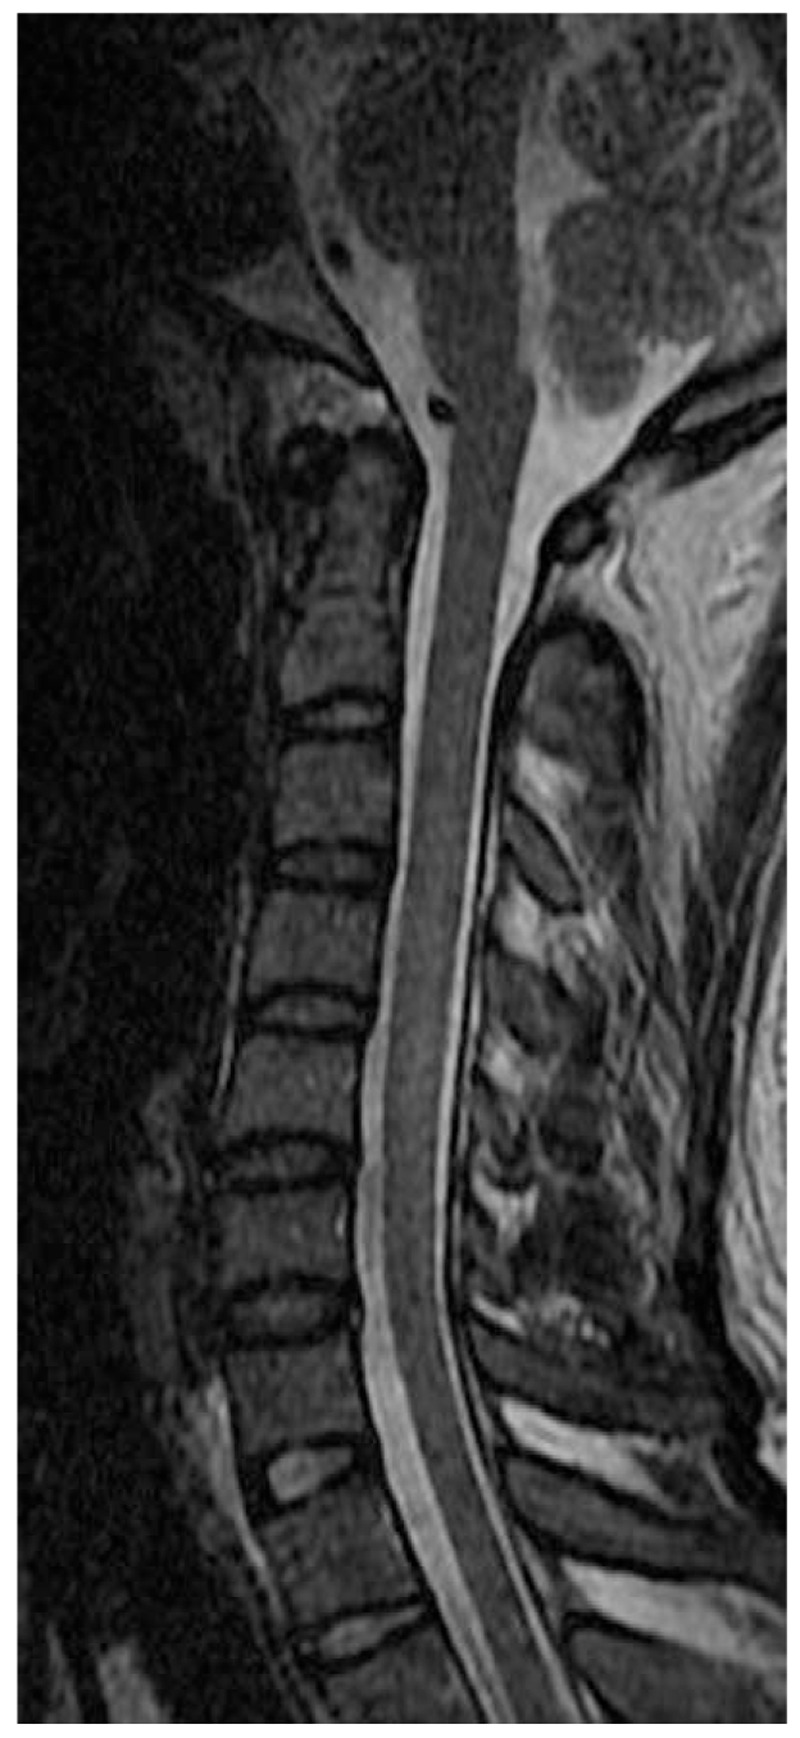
Sagittal magnetic resonance imaging demonstrates increased T2 signal in the posterior columns of the cervical spinal cord from C2 to C6.
